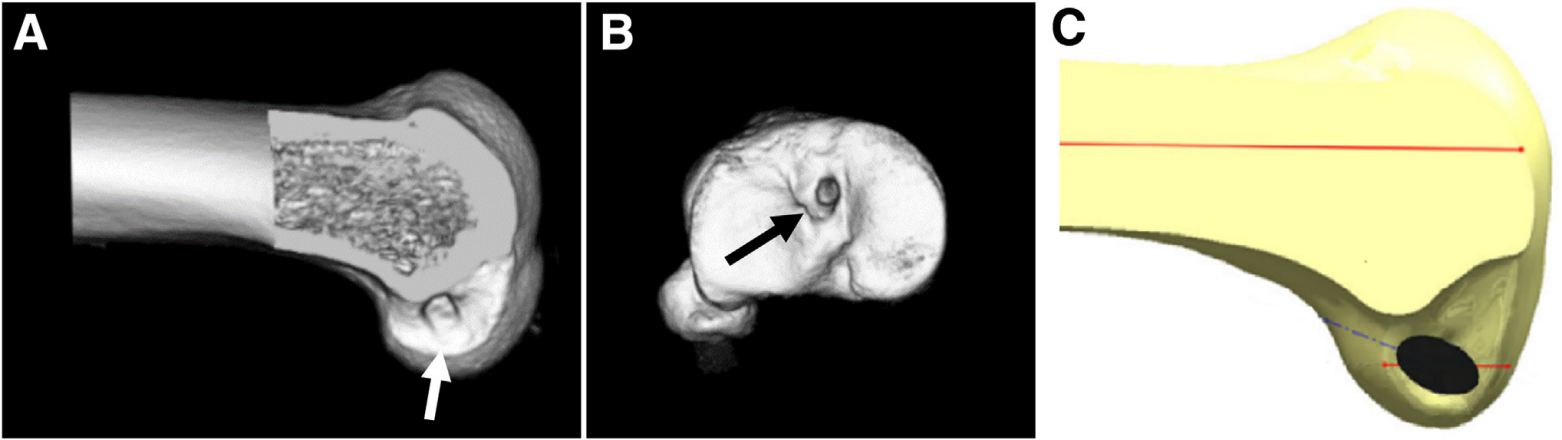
Bone tunnel from the initial surgery in comparison with the anatomic footprint. (A) 3D computed tomography scan of the patient’s left femur showing a circular-shaped femoral tunnel (white arrow) from the initial ACL reconstruction located too anterior. (B) 3D computed tomography scan of the patient’s left tibia showing the tibial tunnel (black arrow) from the initial ACL reconstruction in an anatomic location. (C) Anatomic location of the ACL femoral footprint (black oval) in a left femur. (Adapted from Lubowitz et al.^9^) (3D, 3-dimensional; ACL, anterior cruciate ligament.)
